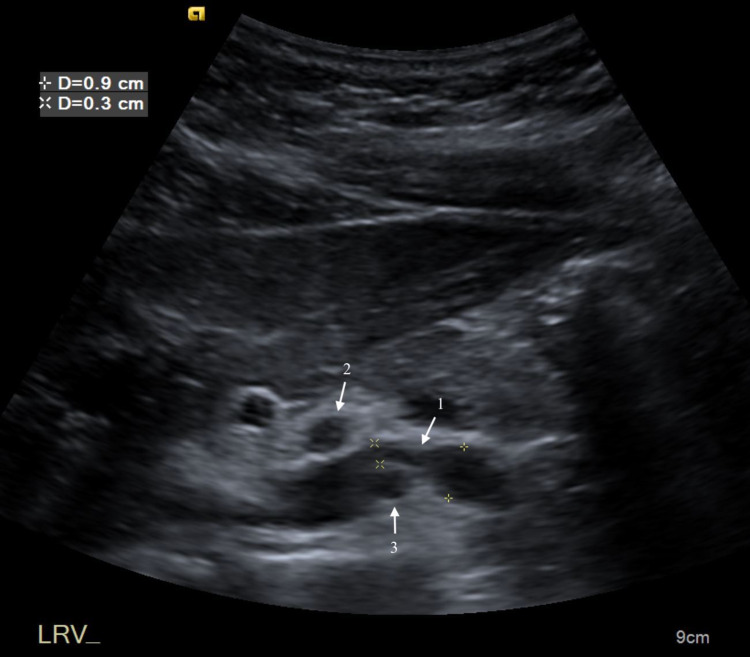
Transverse ultrasound of upper abdomen demonstrates compression of the LRV (1) between SMA (2) and the aorta (3). LRV - left renal vein; SMA - superior mesenteric artery
